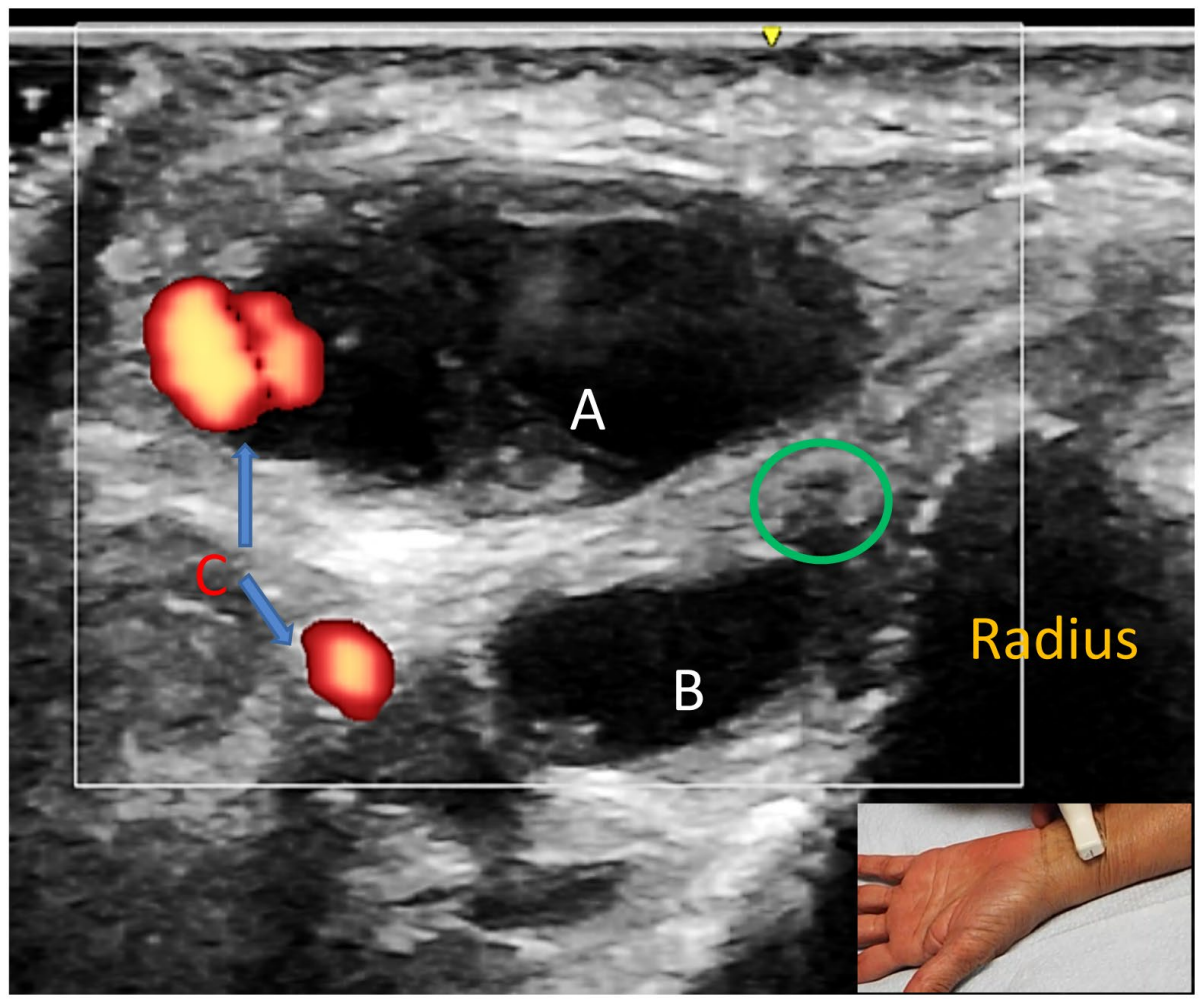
Case 33: Short axis view proximal to the wrist showing bilobed cyst (A,B) and the superficial radial nerve (green circle). Color Doppler shows blood vessels (orange) close to the cyst (C).
